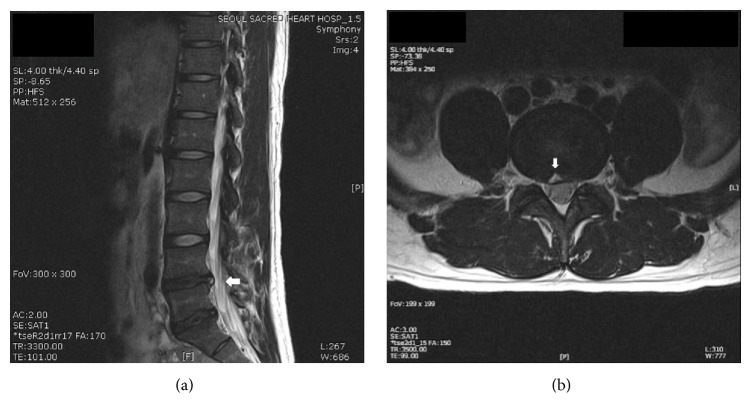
A T2-weighted magnetic resonance image showing the high-intensity zone (arrow) in a patient with a L4-5 herniated lumbar intervertebral disc. Sagittal view (a) and axial view (b).
